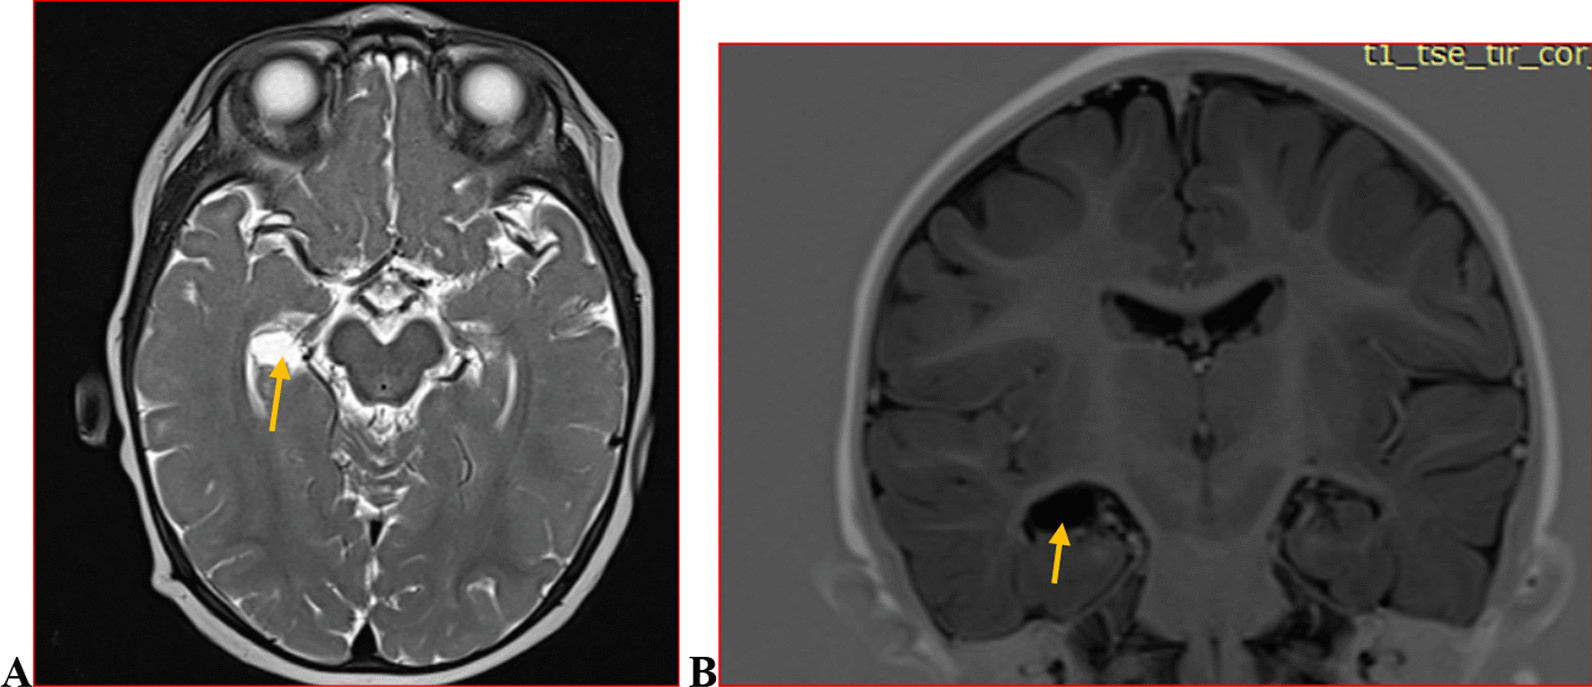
Axial T2 (A) and coronal T1 weighted (B) MRI demonstrating a well defined cyst with CSF signal intensity on all sequences in the plane of the right choroidal fissure (arrow) compressing the ipsilateral hippocampal head
